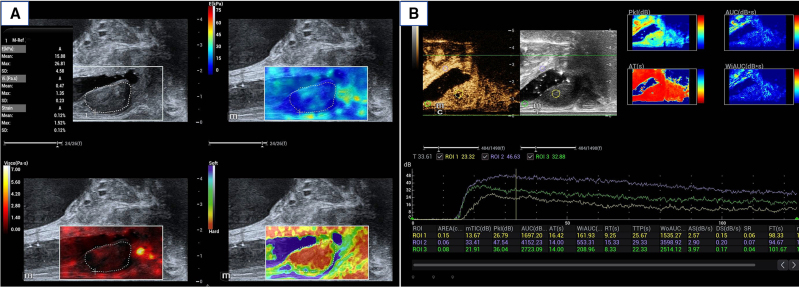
Multi-parameter combined imaging and CEUS images. (A) Shear wave elastography in the upper right, viscoelastic imaging in the lower left, and strain elastography in the lower right; (B) CEUS time-intensity curve (TIC). CEUS = contrast-enhanced ultrasound.
